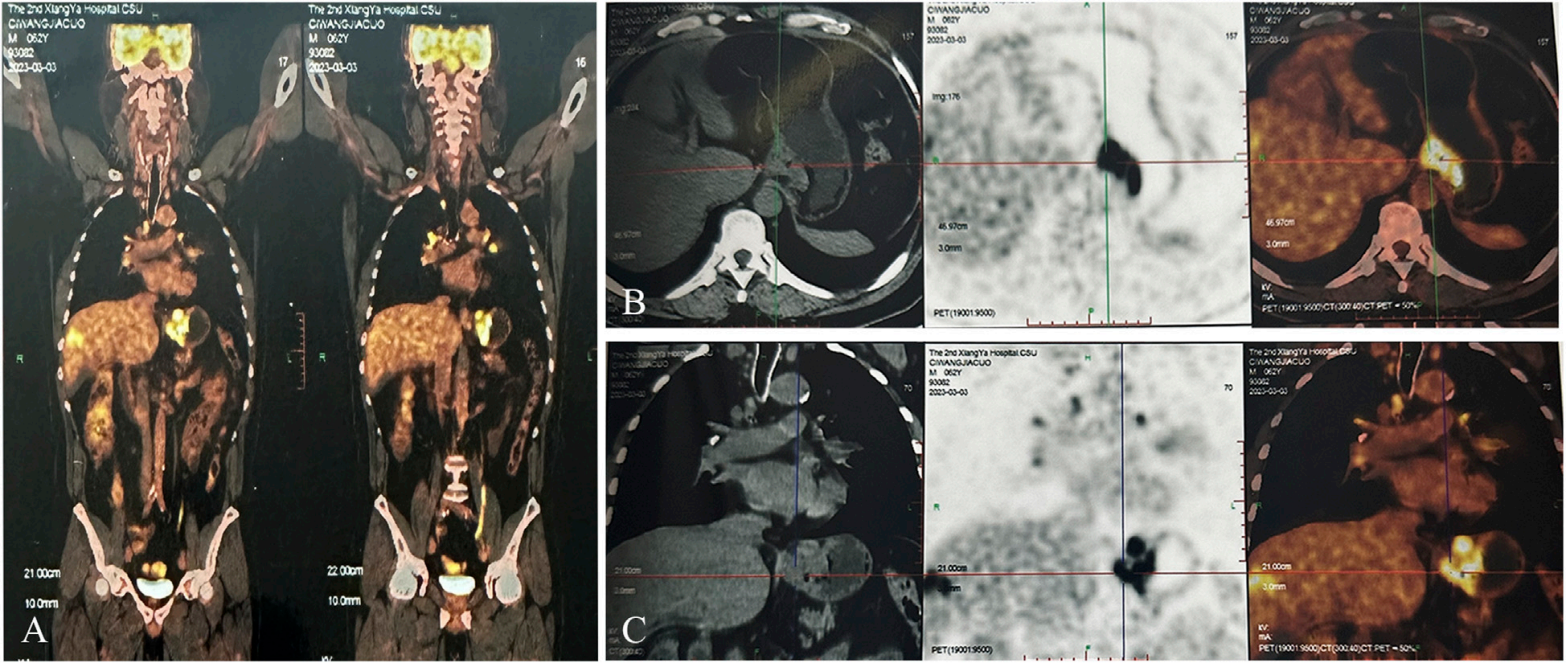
PET-CT examination before initial anti-tumor treatment, considering gastric cardia cancer with peripheral lymph nodes and liver metastasis. (A) Overall image. (B) Cross-sectional image. (C) Coronal image).
